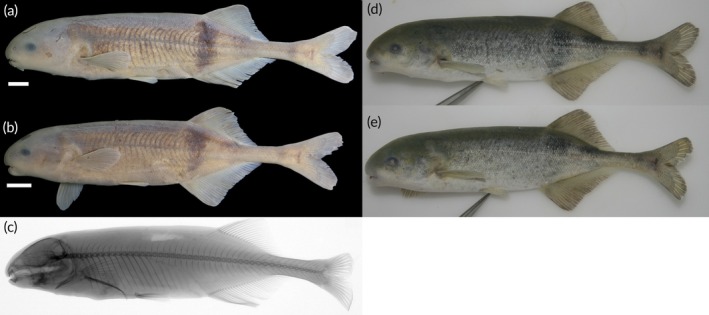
(a) Holotype of *Heteromormyrus angusticaudata* sp. nov. (SAIAB 85039), 152.5 mm standard length (SL), from the Kwanza River; (b) paratype of *H. angusticaudata* sp. nov. (SAIAB 85039), 149.0 mm SL, from the Kwanza River, southern Africa; (c) X‐ray radiograph of holotype; (d, e) live pictures of *H. angusticaudata* sp. nov. Scale bar =1 cm.
